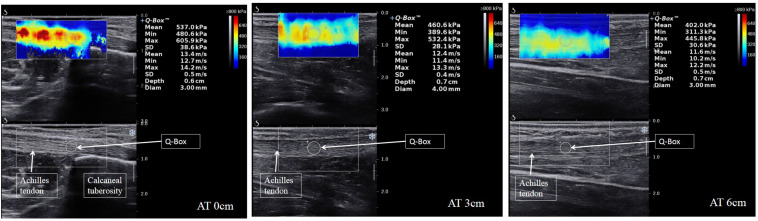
Longitudinal shear wave elastography images of the three regions of the AT in patients with PF show the values of the Young’s modulus at a relaxed ankle position. The Q-Box^TM^ is shown on the right of each scale sonogram.
